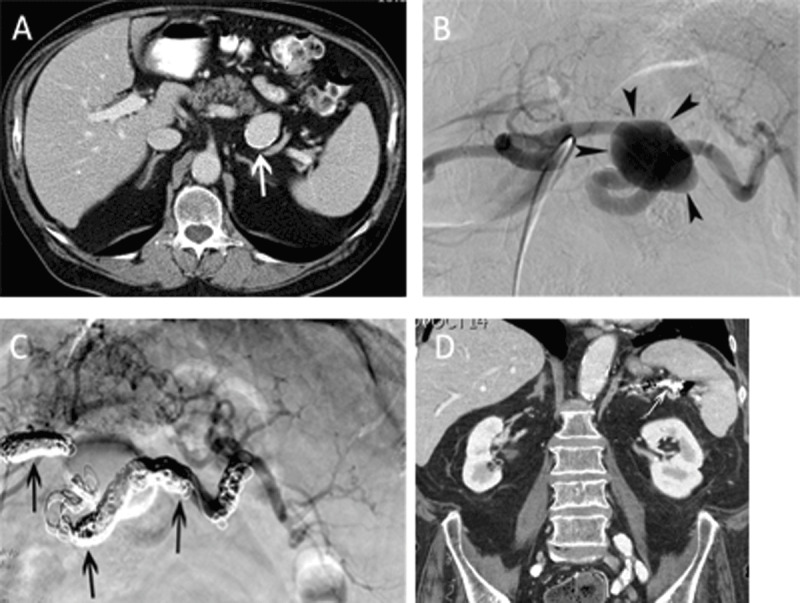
Main splenic artery embolization for atherosclerotic aneurysm. (A) Contrast-enhanced CT-scan in a 74-year-old man revealed an asymptomatic, atherosclerotic aneurysm (white arrow) with a maximal diameter of 37 mm. The total splenic volume was 418 ml. (B) Selective angiography of the celiac trunk confirmed the saccular aneurysm (arrowheads) in the middle third of the main splenic artery. (C) Completion angiography after coil embolization (arrows) demonstrated exclusion of the aneurysm and reinjection of the intrasplenic arteries through gastric collaterals. (D) Follow-up CT-scan 5 years after coil embolization revealed an homogeneously enhancing spleen with a total volume of 264 ml.
